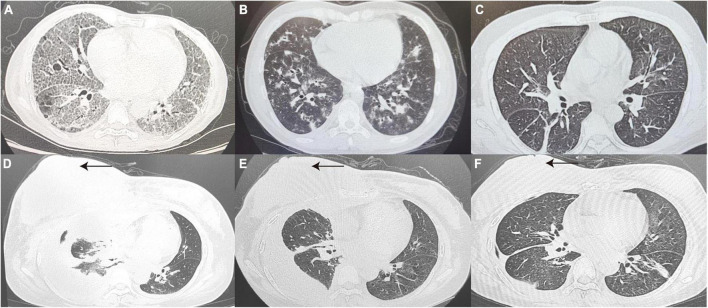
True-negative representative cases. (A) A 41 year old woman diagnosed with pulmonary alveolar proteinosis, CT Scan revealed thickened interlobular septa and multiple patchy with a reticular pattern changes in the subpleural of Bilateral lungs. (B) A 32 year old post-transplant AML patient with a final diagnosis of IPS, CT Scan revealed diffuse distribution of flocculent hyperdense shadows in Bilateral lungs, with some lesions fused into a sheet. (C) Chest CT Images confirmed drug-induced interstitial lung disease, showing interstitial changes in Bilateral lungs with disorganized broncho-vascular bundles and interlobular septal thickening with a reticular pattern. (D–F) Patient with relapsed DLBCL presented with chest tightness and pleural effusion on CT, 7 days after infusion of anti-CD19 CAR T-cells (D). At the same time, patient’s peripheral blood CAR T-cell copy number, IL-6 and ferritin were elevated, consideration of CAR T-cell-associated pulmonary toxicity, patient lung lesions improved 1 month after tolimumab and chest fluid drainage (E). A review more than 3 months later showed right breast lesion (as indicated by the black arrow) and lung lesion were significantly better than before (F). CT, computed tomography; AML, acute myeloid leukemia; DLBCL, diffuse large B-cell lymphoma; IL-6, interleukin-6.
